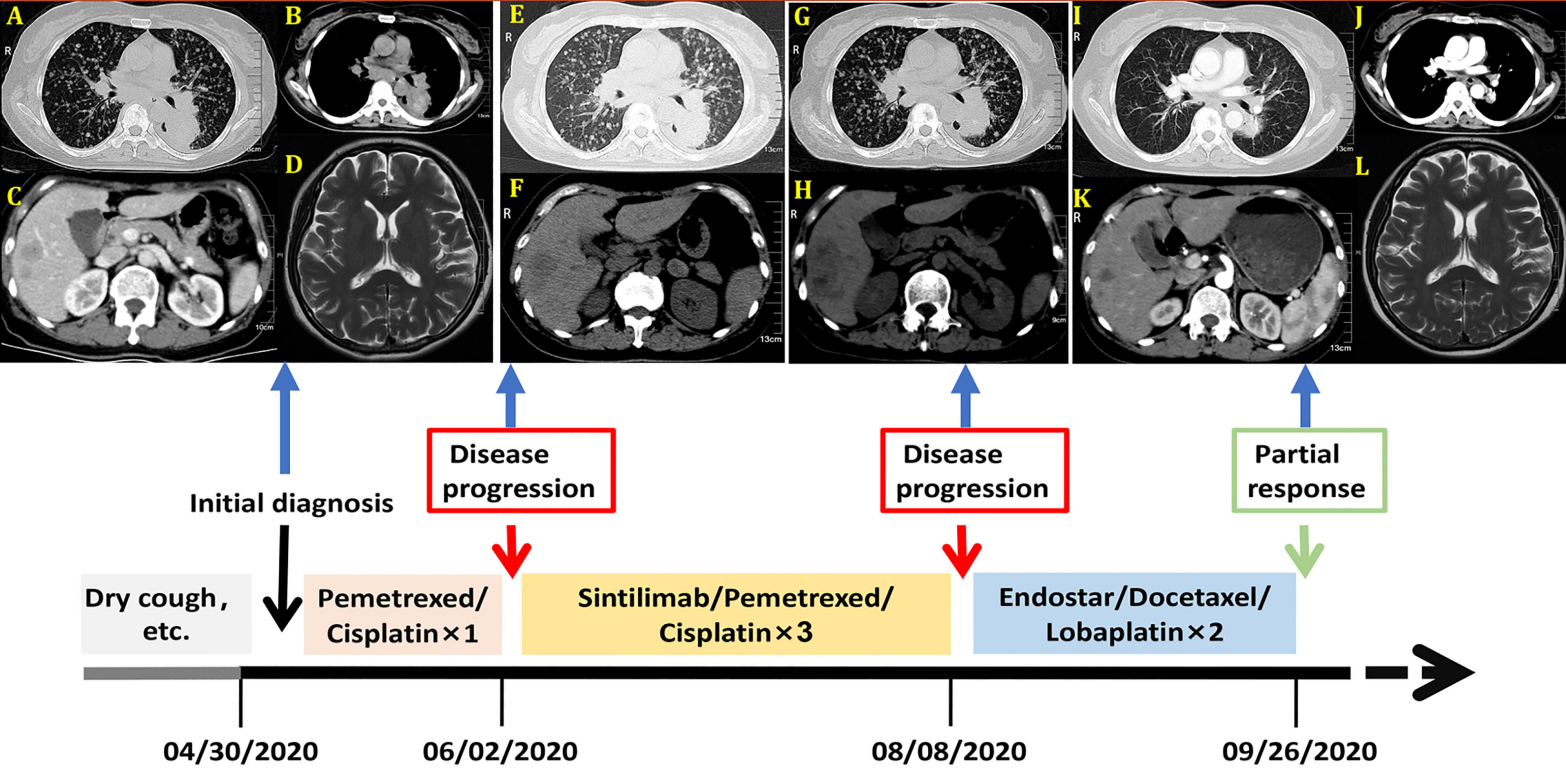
Imagines of computed tomography (CT) and Magnetic resonance (MR) before treatment. (A) Chest CT scan with lung window; (B) Chest CT scan with mediastinal window; (C) Abdominal-enhanced CT scan; (D) Head magnetic resonance (MR) image. Imagines of CT after the first cycle of pemetrexed and cisplatin. (E) Chest CT scan with lung window; (F) Abdominal CT scan; Imagines of CT after three cycles of Sintilimab combined with pemetrexed and cisplatin. (G) Chest CT scan with lung window; (H) Abdominal CT scan; Imagines of computed tomography (CT) and Magnetic resonance (MR) after two cycles of Endostar combined with docetaxel and lobaplatin. (I) Chest CT scan with lung window; (J) Chest CT scan with mediastinal window; (K) Abdominal-enhanced CT scan; (L) Head magnetic resonance (MR) image. These imagines were showed the timeline of different treatments for the patient’s entire treatment progression.
